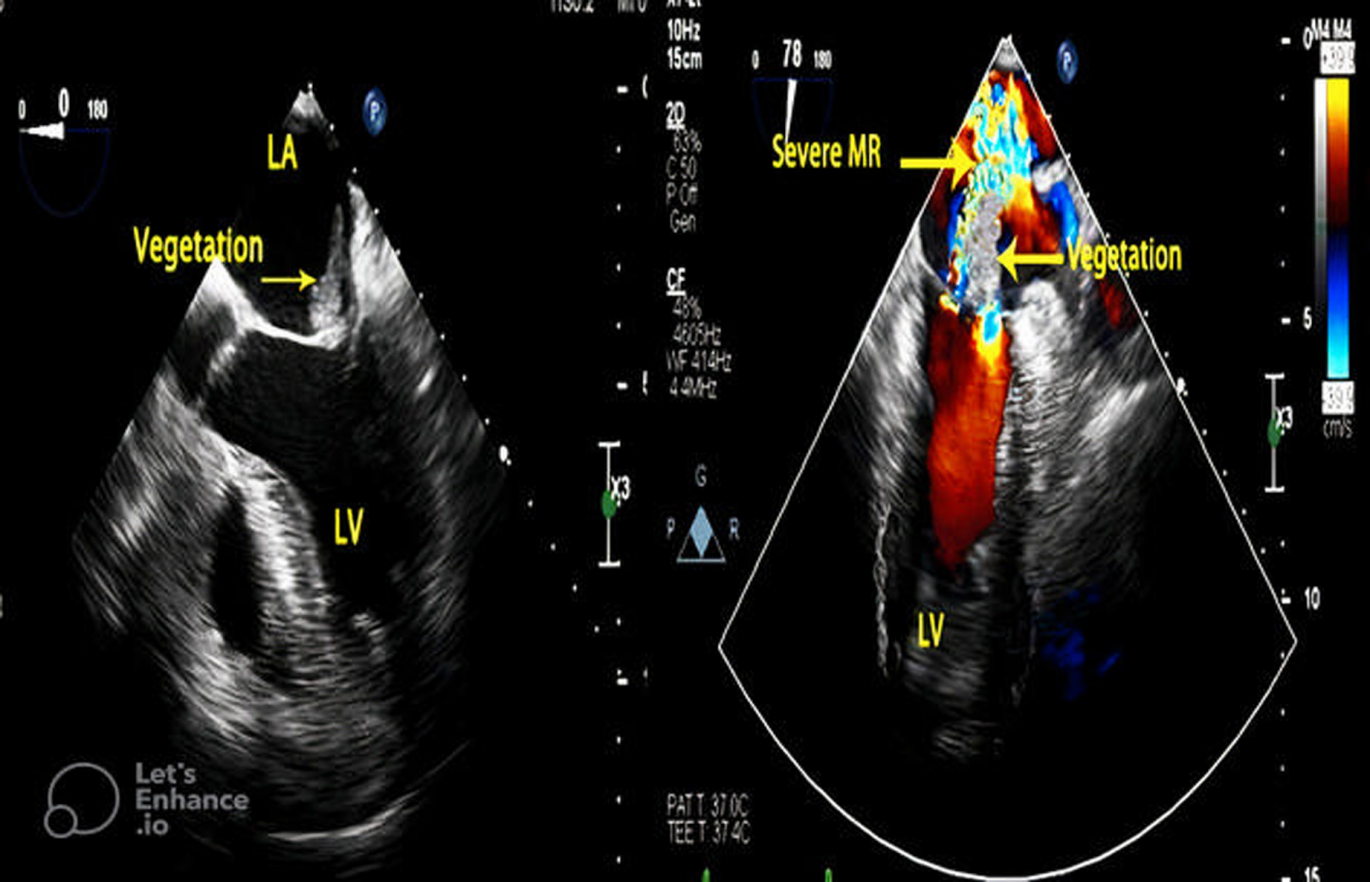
Transesophageal echocardiography in mid‐esophageal view (0 degree) showed large vegetation attached to the atrial side of anterior mitral valve leaflet (A), and Transesophageal two‐dimensional echocardiography and color Doppler flow velocity mapping in the mid‐esophageal long‐axis view (78 degree) revealed severe MR due to infective destruction of the anterior mitral leaflet (B). LA, left atrium; LV, left ventricle; MR, mitral regurgitation
